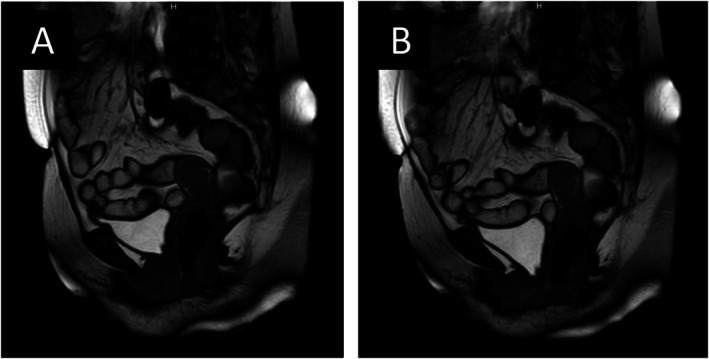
Cine magnetic resonance imaging (MRI) images depict the position of pelvic organs after pessary removal. No pre‐insertion MRI data were available. (A) Cine MRI at rest. (B) Cine MRI with maximum abdominal pressure.
